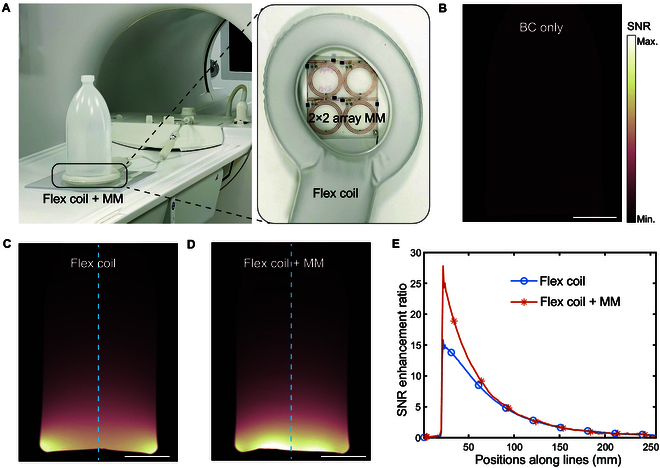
MRI validations for the surface coil enhanced by the metamaterial. (A) Experimental setups. Inset: Configuration of surface coil integrated with the metamaterial. (B) SNR images captured by the BC only. (C) SNR images captured by the surface coil only. (D) SNR image captured by the surface coil combined with the metamaterial. (E) SNR enhancement ratio along blue dashed lines in (C) and (D). Scale bars, 5 cm (B to D).
